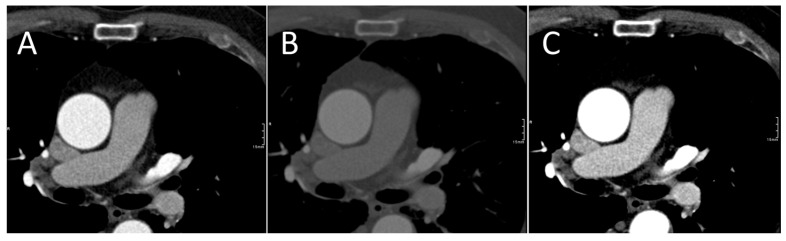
Axial images from an arterial CTA demonstrate the difficulty of clearly delineating the aortic wall in standard CTA windowing (W:1500, L:450) (A), bone windowing (W:2000, L:500) (B) and soft-tissue windowing (W:350, L:50) (C).
